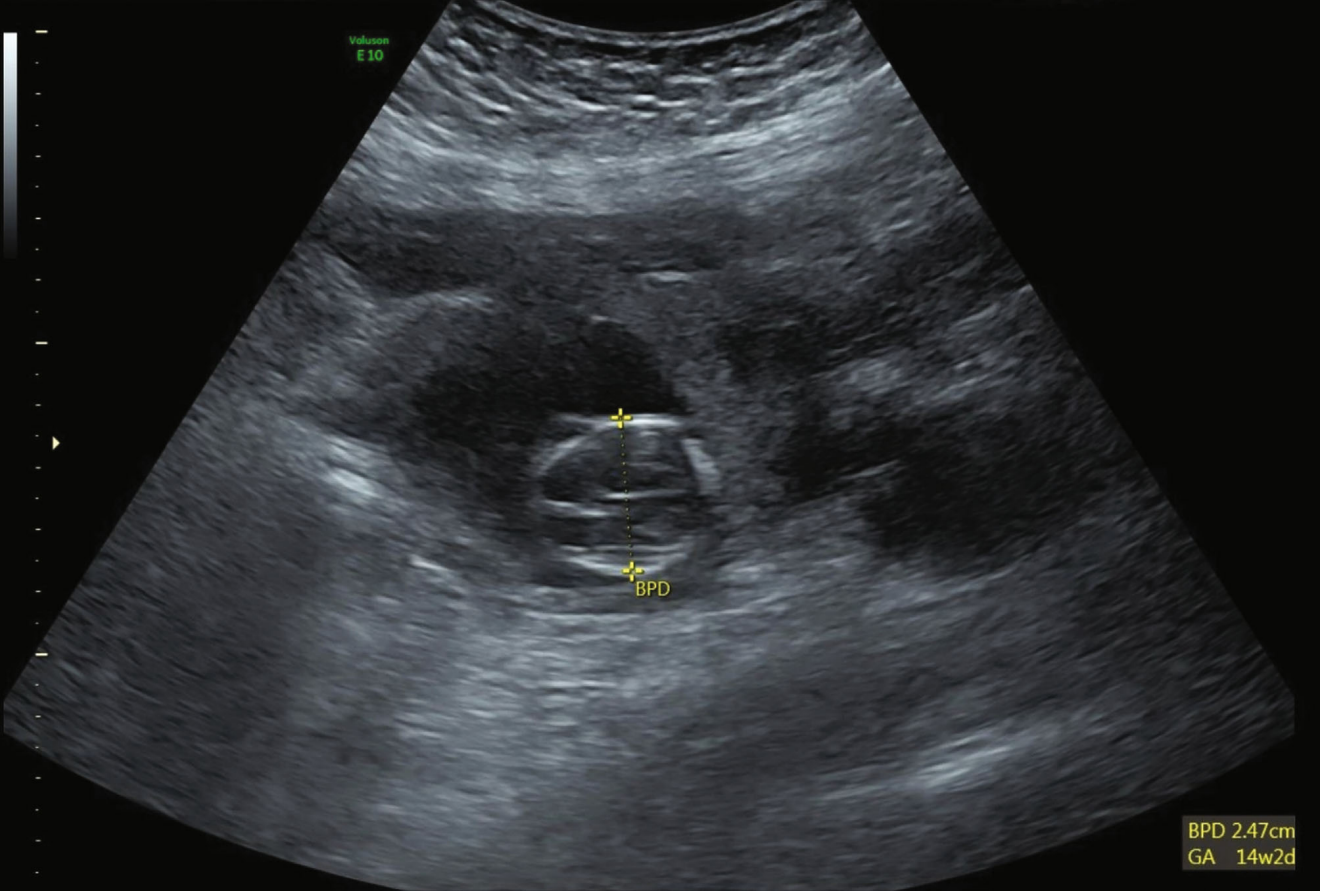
Ultrasound shows the biparietal diameter (BPD) measuring 2.47 cm which equals 14 weeks and 2 days.
